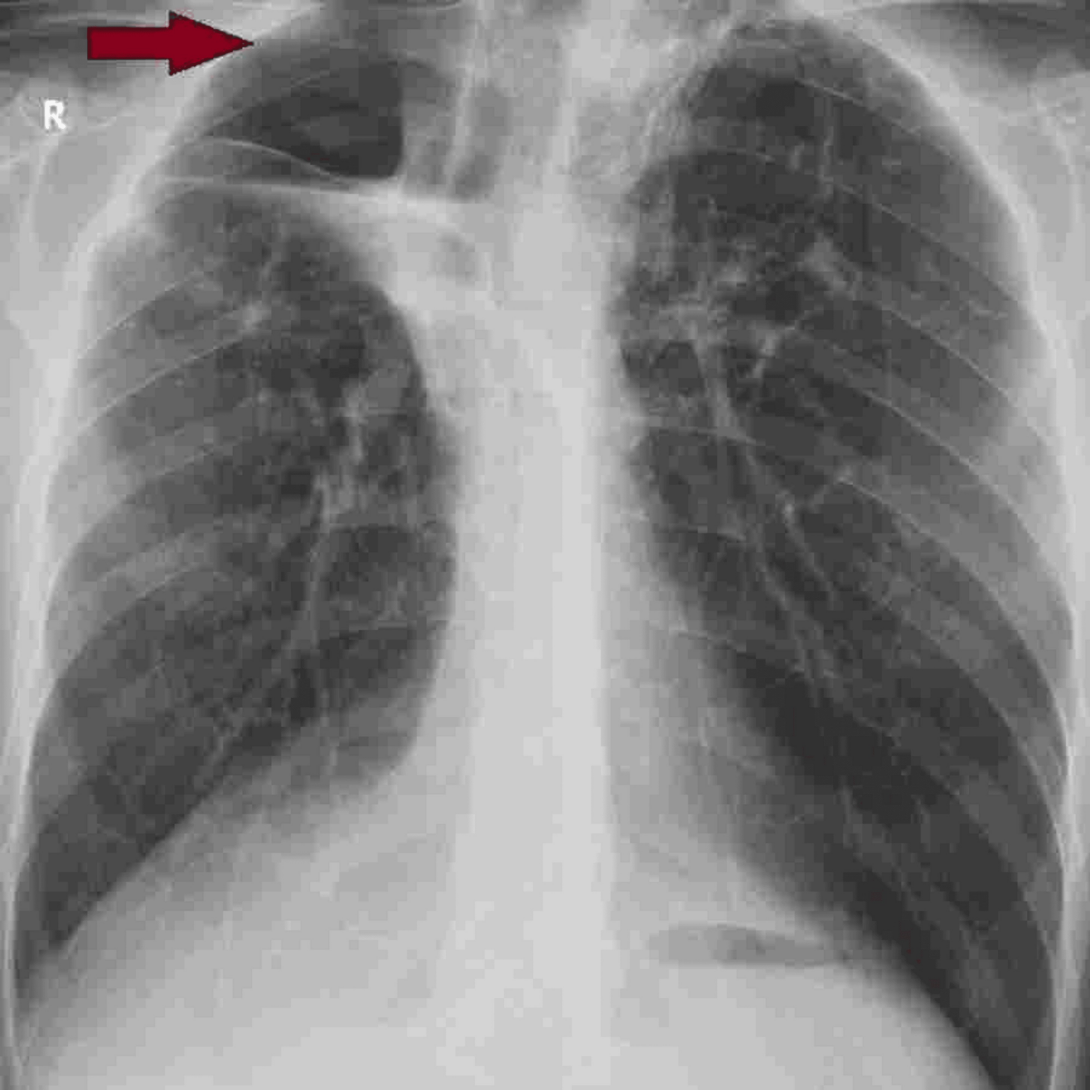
Posterior - anterior view of the chest X-ray one month postoperatively – Expanded lungs with a right apical cavity showing minimal fluid levels - red arrow.
